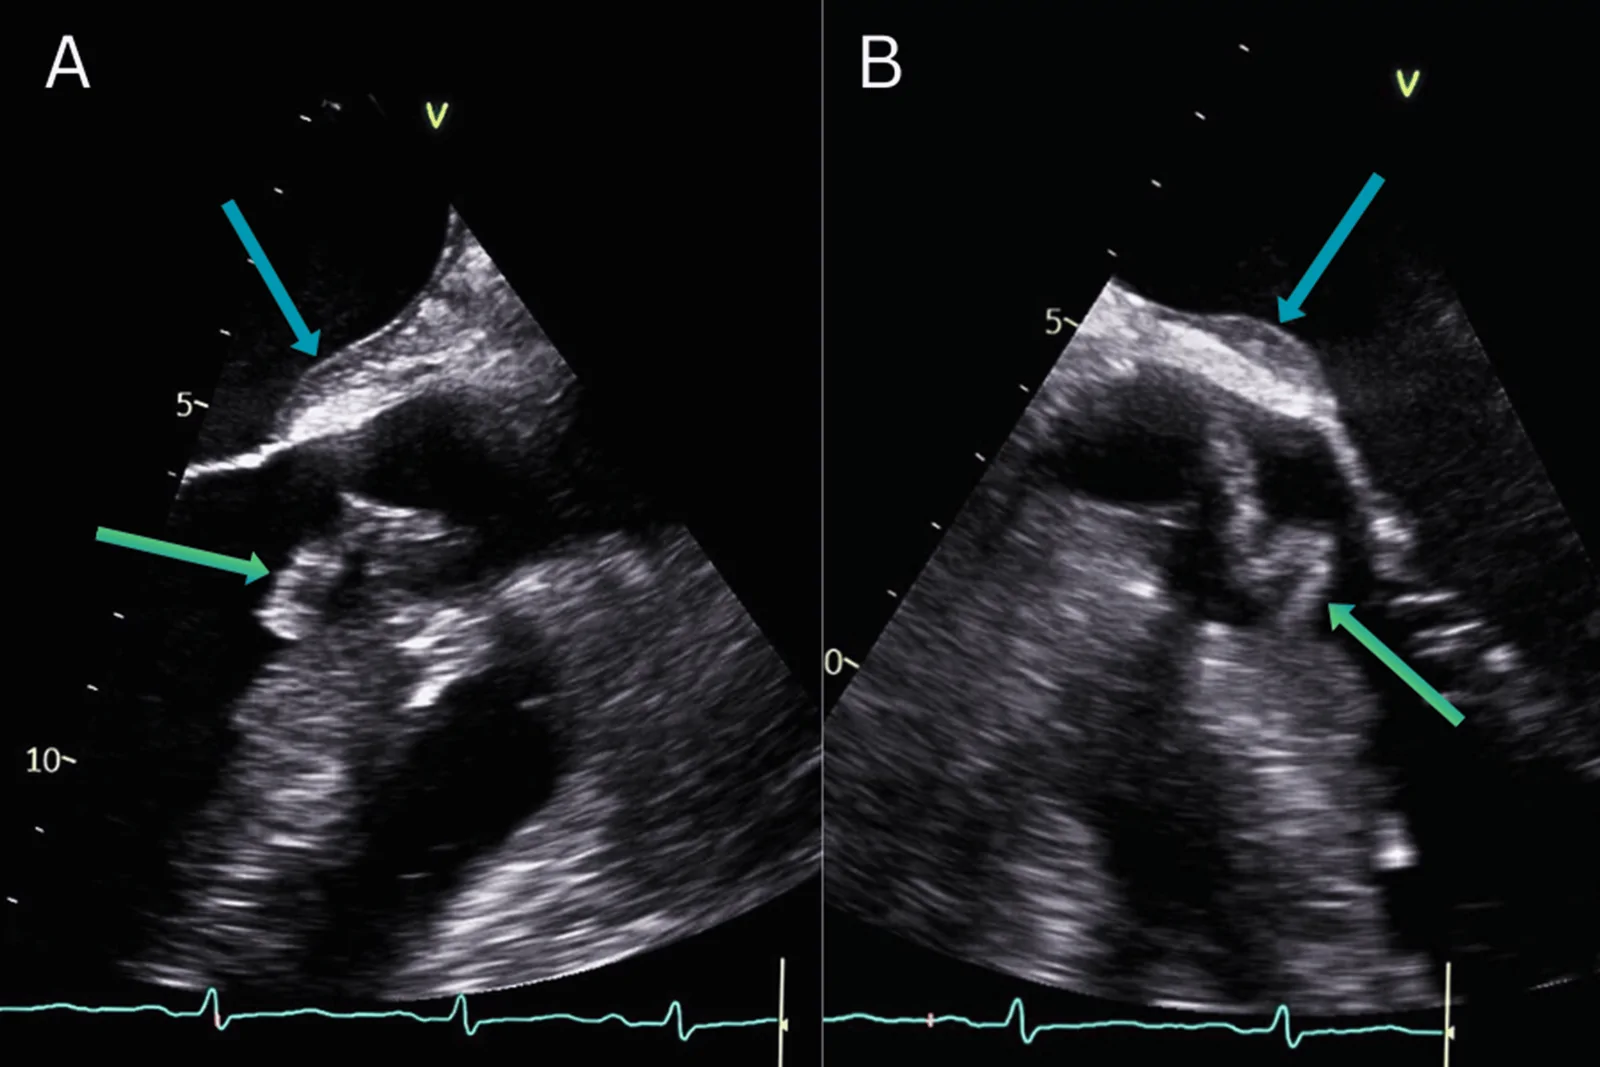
Transesophageal echocardiogram: (A) mid-esophageal view (130°) and (B) mid-esophageal view (0°) demonstrating a heterogeneous echolucent appearance of the aortic root, suggesting a paravalvular abscess (blue arrows) and vegetation on the cusp of the aortic valve (green arrows)
